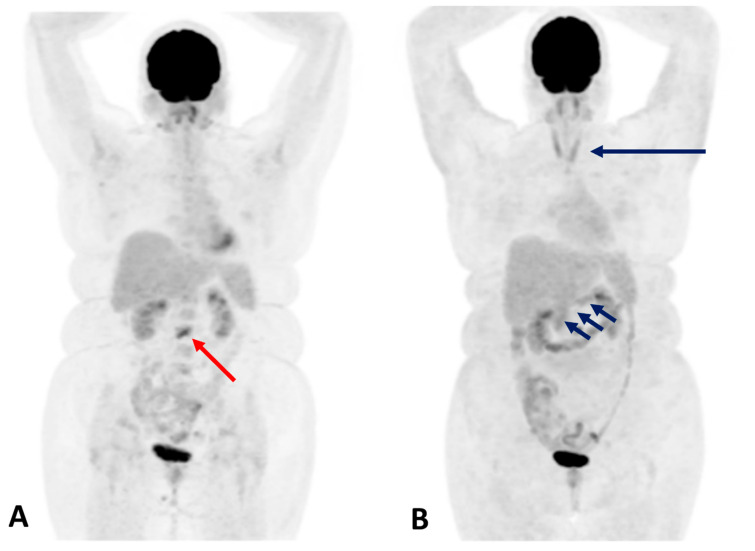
A 53-year-old woman with non-small cell lung cancer underwent surgery, chemotherapy, and radiation therapy for metastatic disease. She subsequently received nivolumab for metastatic progression, most prominently affecting the L2 vertebral body ((A), red arrow). Pre-immunotherapy FDG PET/CT demonstrated no abnormal uptake in the pancreas and thyroid. After four months of therapy, she developed abdominal pain and elevated serum amylase (145 UI/L, normal range: 28–100 UI/L). Follow-up ^18^F-FDG PET/CT revealed stable skeletal metastases and new diffuse increased uptake within both the pancreas and thyroid gland ((B), blue arrows), consistent with immune-related pancreatitis and thyroiditis. Notably, TSH levels remained within normal limits. Following the temporary discontinuation of immunotherapy and conservative management, the patient’s symptoms improved, and serum amylase levels normalized. Sequential ^18^F-FDG PET/CT imaging can monitor response to corticosteroid therapy, with a decreasing uptake trend indicating resolution of immune-mediated pancreatitis. Importantly, focal intense pancreatic uptake may mimic metastatic disease, underscoring the necessity for careful interpretation within the broader clinical, biochemical, and imaging context [49]. Recognizing characteristic patterns of diffuse or focal ^18^F-FDG uptake in patients receiving ICIs is essential to distinguish immune-mediated pancreatitis from neoplastic or infectious processes, thereby enabling prompt endocrine and gastroenterological evaluation and optimal management of immunotherapy.
